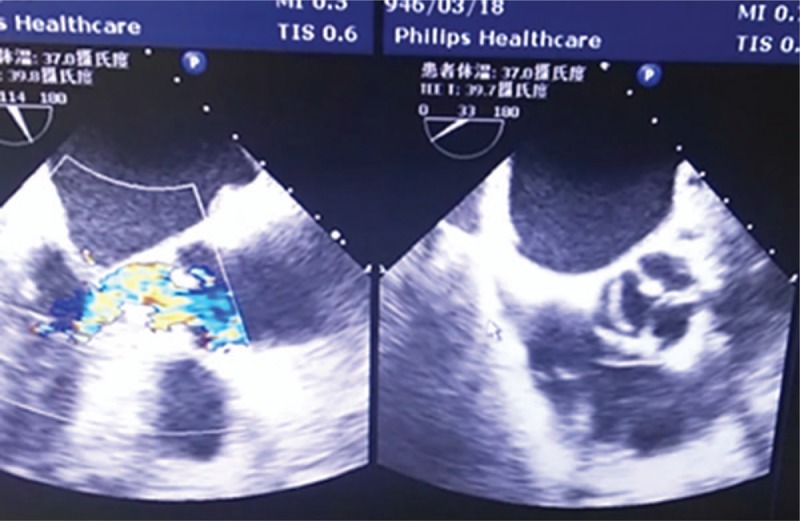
Long axis of transesophageal echocardiography shows hypertrophic obstructive cardiomyopathy with left ventricular outflow tract stenosis of a maximum thickness of 22 mm. The short axis shows that the noncoronary leaflet of aortic valve was well open in the systole, while the left and right coronary leaflets show restricted opening.
